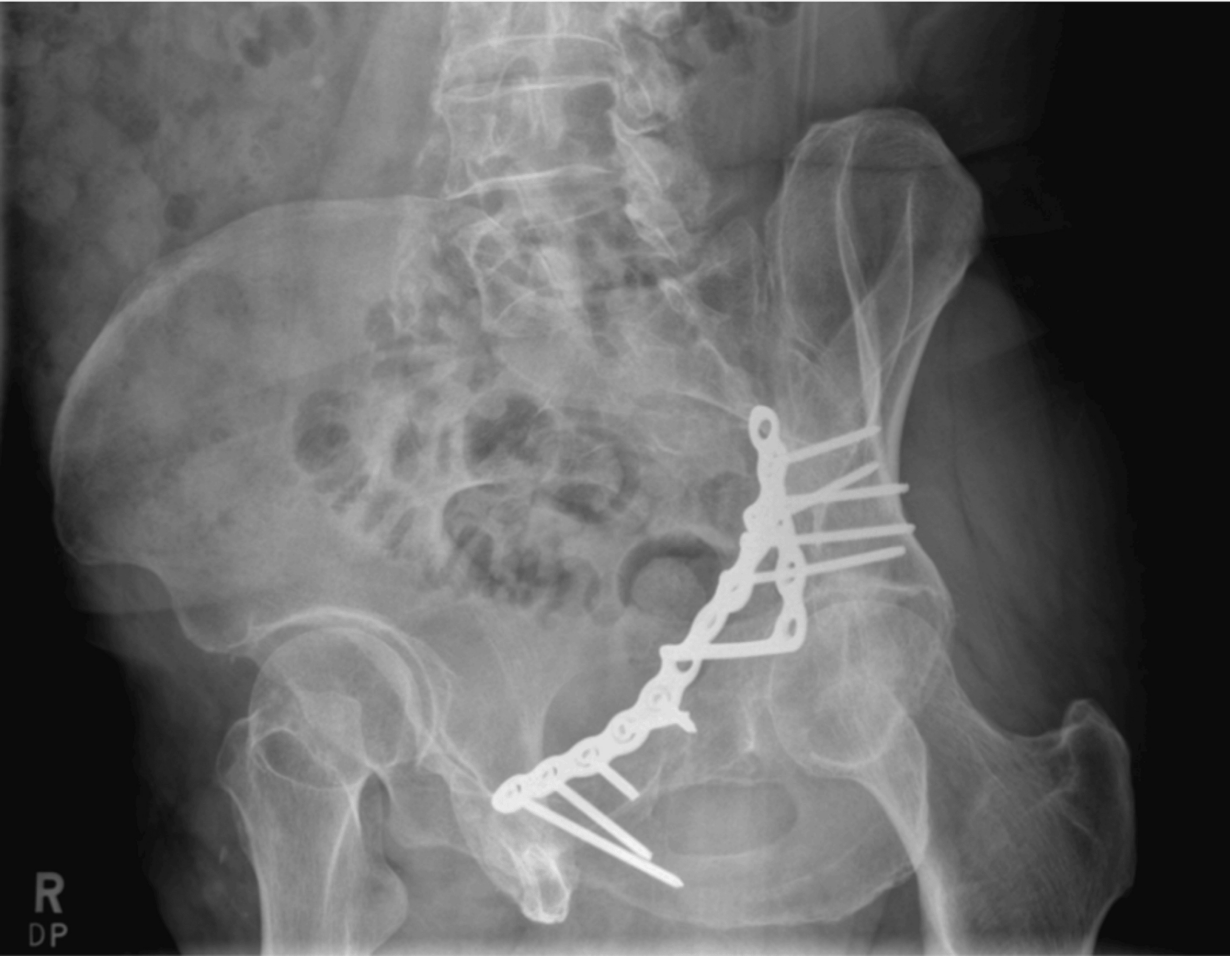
Obturator oblique radiograph of the left hip in a patient, five years following fixation of a displaced acetabular fracture using an anatomical suprapectineal plate
